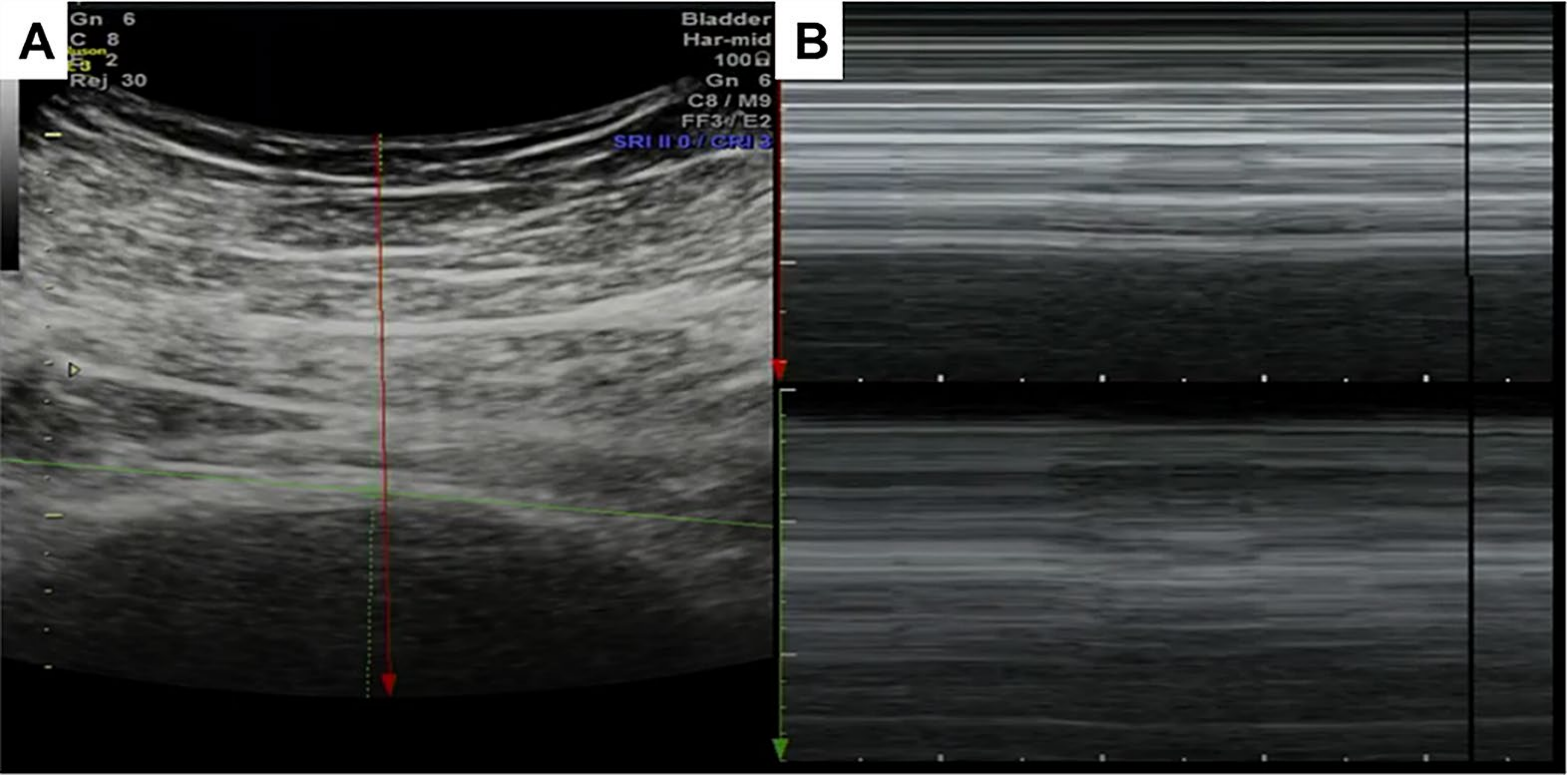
Static images of M mode bladder ultrasound acquired during urodynamic testing during filling. (A) The user manually identifies the outer and luminal bladder wall (green and red lines) and (B) a 1-dimensional bladder wall slice is displayed over time. A post-hoc texture tracking algorithm then records wall thickness over time as a measure of micromotion
